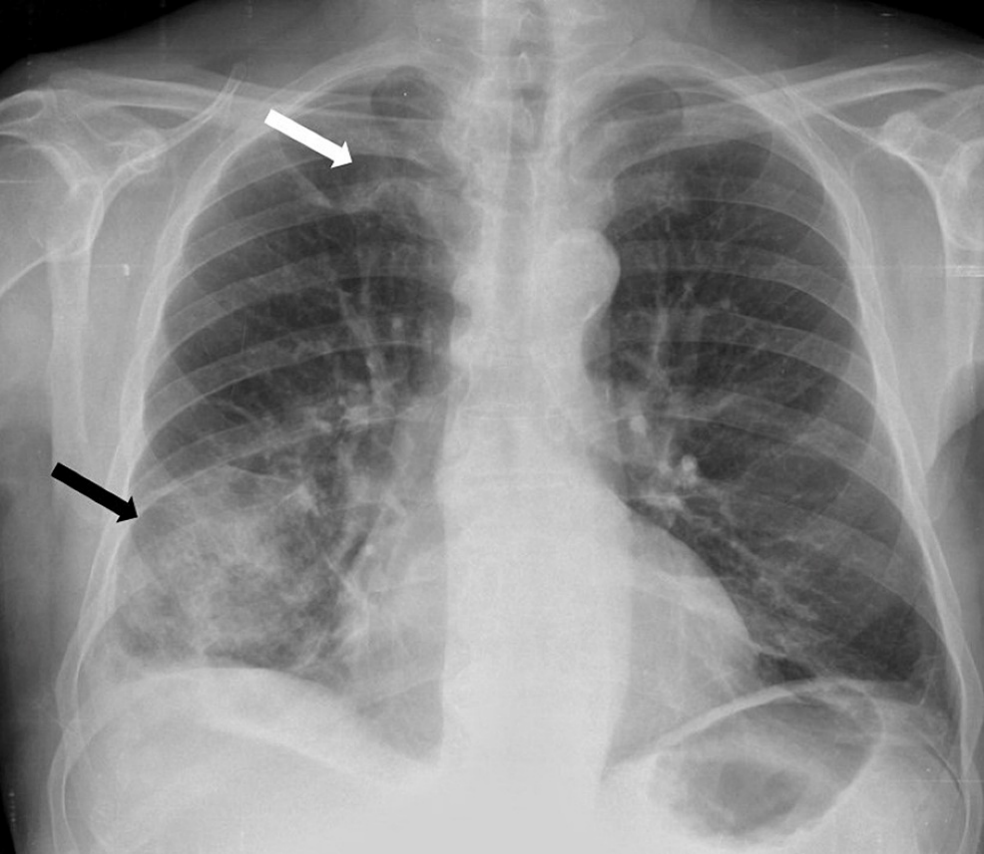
Chest X-ray obtained in the first observation, revealing two images of condensation, in the right pulmonary base (black arrow) and in the superior right lobe (white arrow).
